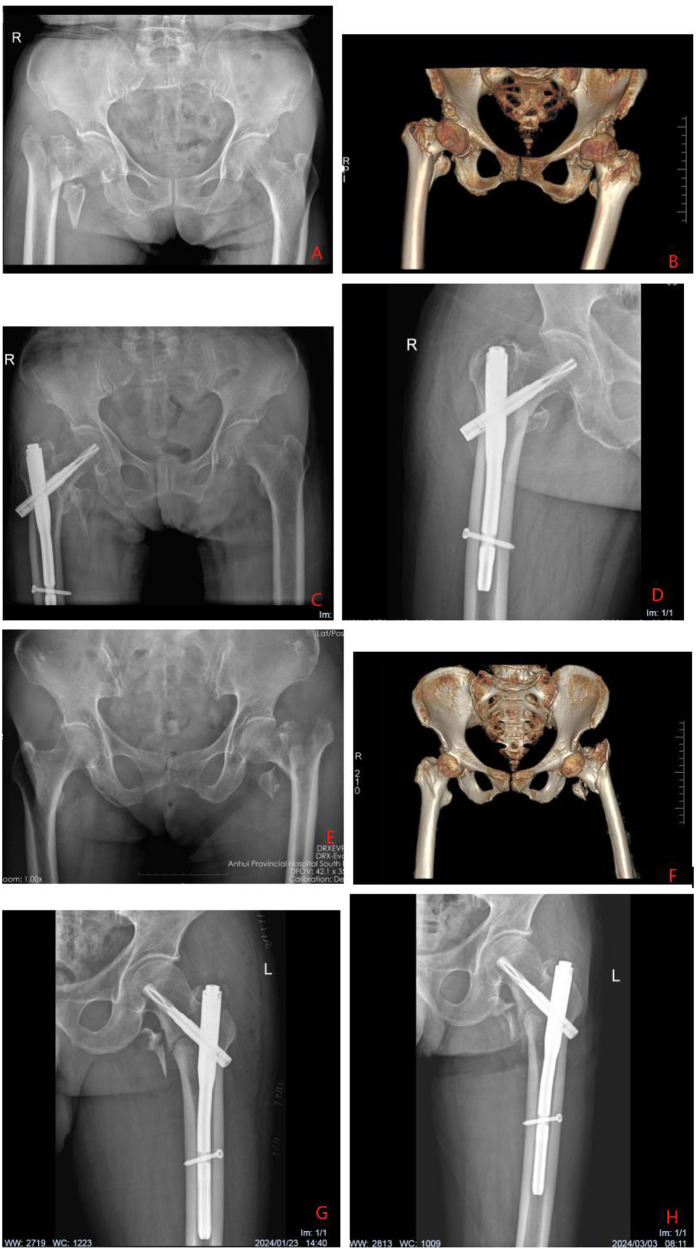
(A–D) Shows a 75 year old male patient with right intertrochanteric fracture. According to ao/ota classification, the fracture is 31a2. Who was treated with clamping and bone traction device. (A) Shows the preoperative anteroposterior radiograph of both hips (1:15 cm), (B) shows the preoperative three-dimensional reconstruction radiograph of both hips (1:20 cm), (C) shows the anteroposterior radiograph of both hips (1:15 cm) on the first day after operation, and (D) shows the anteroposterior radiograph of the right hip (1:10 cm) on the third month after operation. (E–H) Shows a 75 year old male patient with left intertrochanteric fracture. The ao/ota classification is 31a2. Who was treated with clamping and traction bed. (A) Shows the preoperative anteroposterior radiograph of both hips (1:15 cm), (B) shows the preoperative three-dimensional reconstruction radiograph of both hips (1:20 cm), (C) shows the anteroposterior radiograph of the left hip on the day after operation (1:10 cm), and (D) shows the anteroposterior radiograph of the left hip on the third month after operation (1:10 cm).
